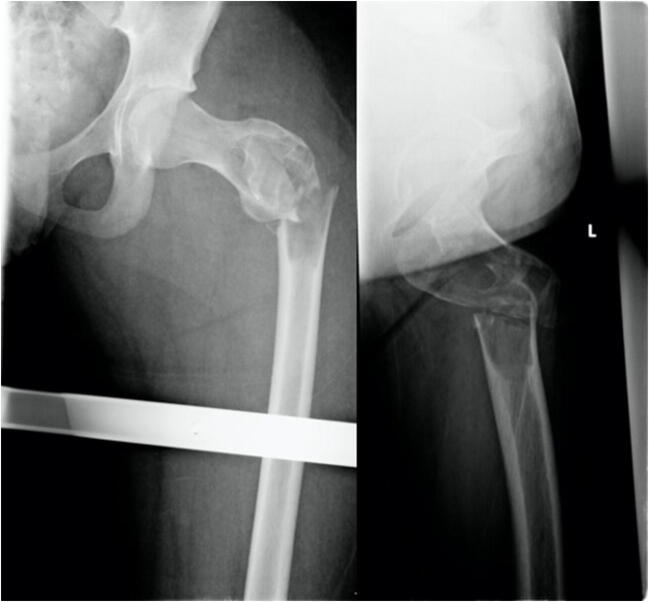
Anterior-posterior and axial X-ray of a displaced trochanteric fracture in a 16-year-old boy with a bone cyst. Full shaft displacement and an axis deviation of 70°
